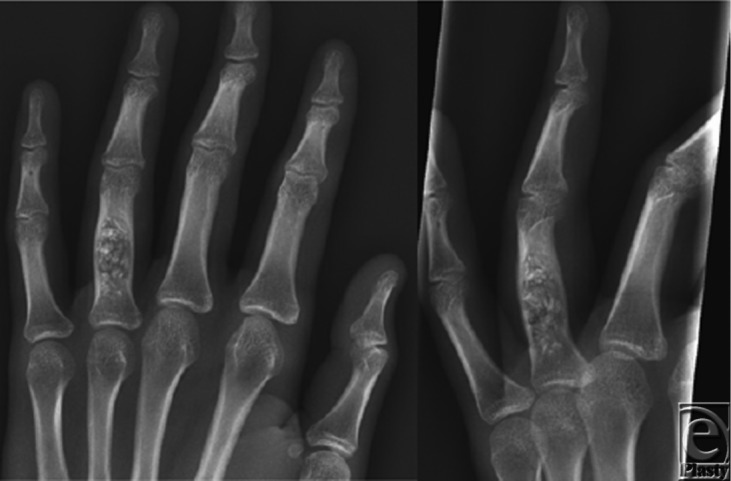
Radiographs of the left hand. Two months' interval from the time of curettage and bone grafting of ring finger midshaft proximal phalanx. There is no pathological fracture or new lytic lesion.
